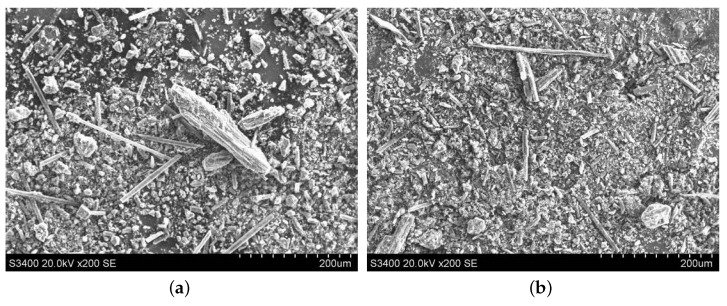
Morphologies comparison when $a_{p}$ is 0.2 mm. (a) is ordinary cutting and (b) is ultrasound- assisted cutting.
